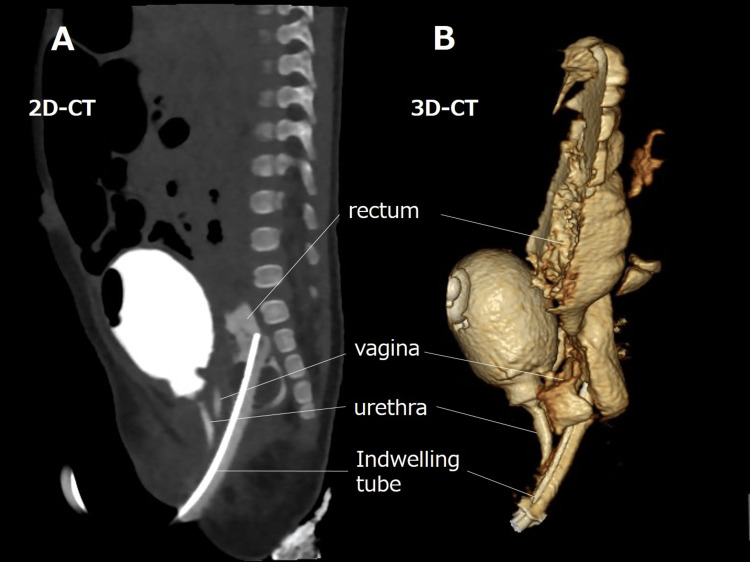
Preoperative imaging of cloacal malformation (A) Sagittal 2D CT cloacagram showing the dilated common channel and the transanally indwelling drainage tube. (B) 3D reconstructed CT cloacagram clearly visualizing the rectum, vagina, and urethra converging into the common channel.
